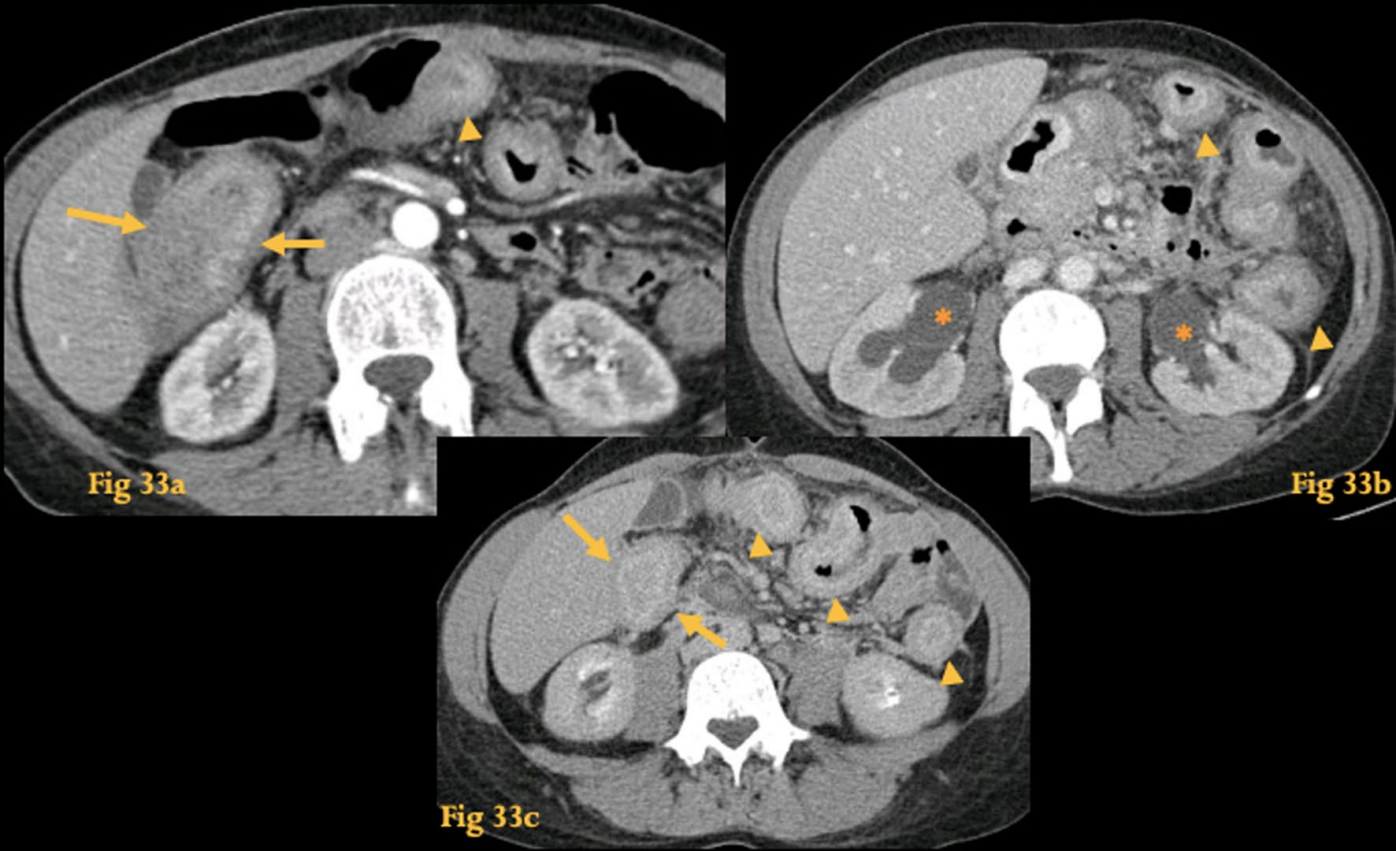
Vasculitis (lupus). Abdominal CT of a patient with systemic lupus erythematosus and antiphospholipid syndrome (a). There is evidence of multifocal thickening of several bowel loops with a target appearance (arrowheads), including the stomach, colon and D2 (arrows). Note also bilateral hydronephrosis (asterisks in b). Abdominal CT (c) of the same patient following 3 weeks of steroid treatment with no clinical improvement revealed resolution of some of the previous affected segments, while other bowel segments showed wall thickening for the first time
